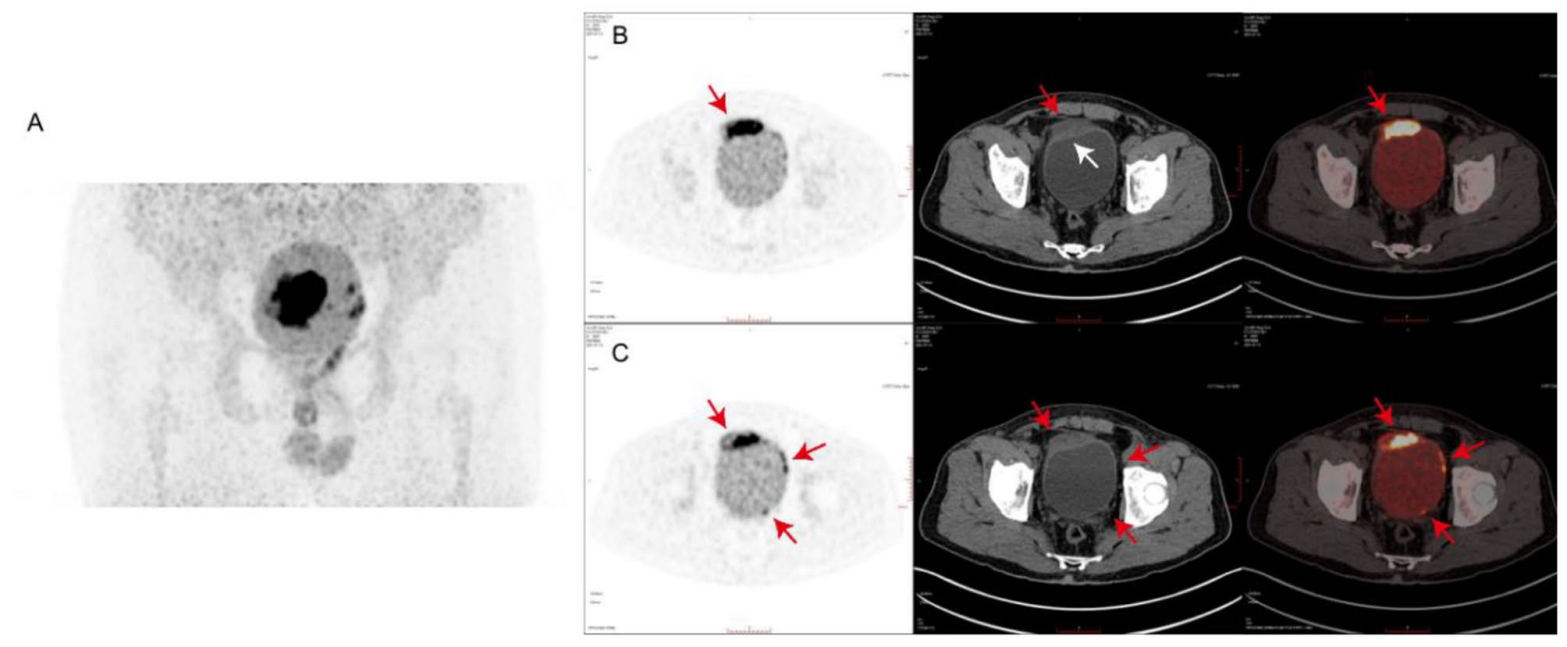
The FDG uptake of the bladder (A), the anterior wall(B), the left anterior and the left posterior (C) wall in 18 F-FDG-PET/CT. The SUVmax was 36.1 in the anterior wall. The SUVmax was 11.5 in the left anterior wall and the left posterior wall. The red arrows show the FDG accumulation sites. The white arrow shows the biopsy site
